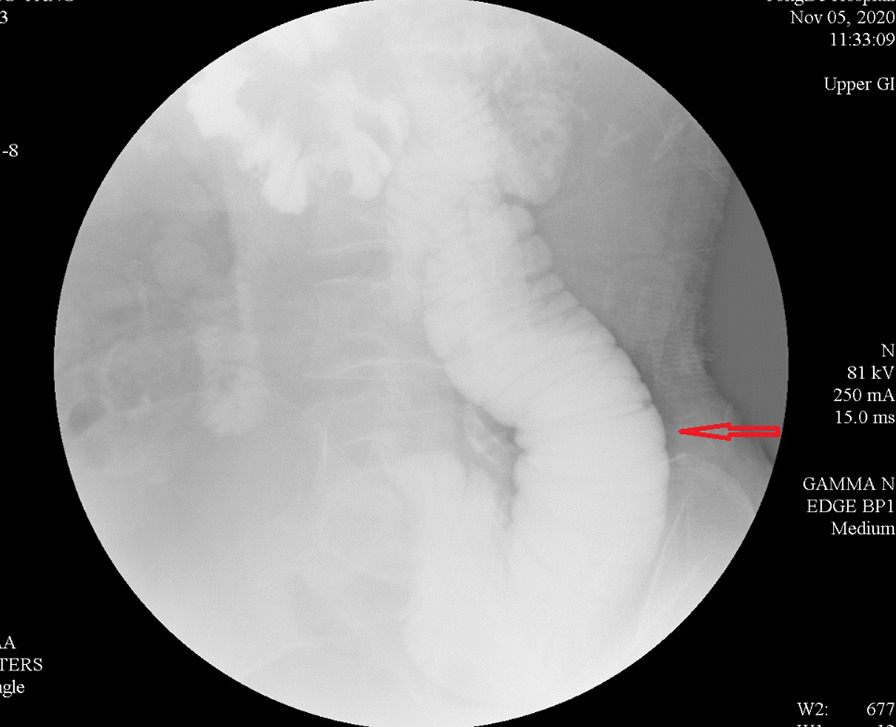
Gastrointestinal radiography suggests small bowel obstruction
